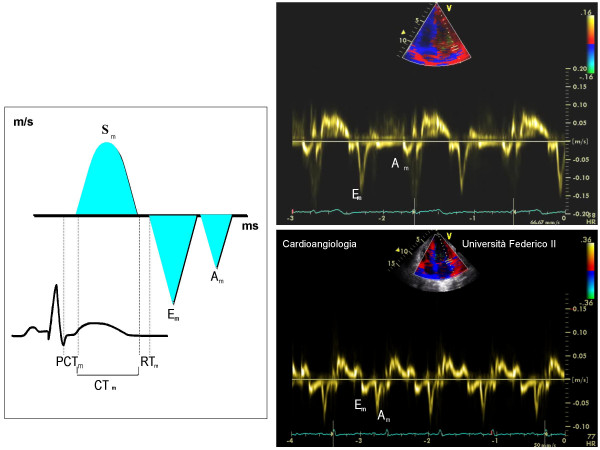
In the left screen, methodological outline for the measurement of Tissue Doppler indexes. In the right screen, normal myocardial diastolic pattern (upper part) and pattern of abnormal myocardial relaxation (lower part). A_m _= myocardial atrial velocity (cm/s), CT_m _= myocardial contraction time (ms), DT_m _= myocardial deceleration time of E_m_(ms), E_m _= myocardial early-diastolic velocity (cm/s), PCT_m _= myocardial pre-contraction time (ms), RT_m _= myocardial relaxation time (ms).
